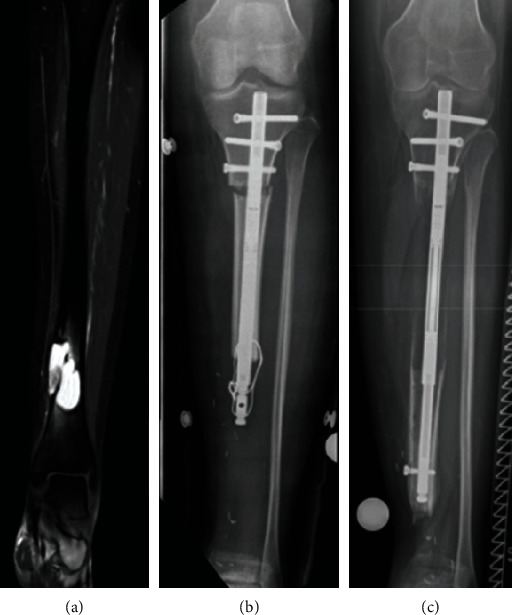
Patient 1. (a) Magnetic resonance imaging showing adamantinoma of the distal tibia. (b) Radiograph showing wide tumor resection preserving the fibula, proximal tibial corticotomy, and implantation of a lengthening nail for bone segment transport. (c) Radiograph showing advanced bone segment transport after exchange to another lengthening nail.
